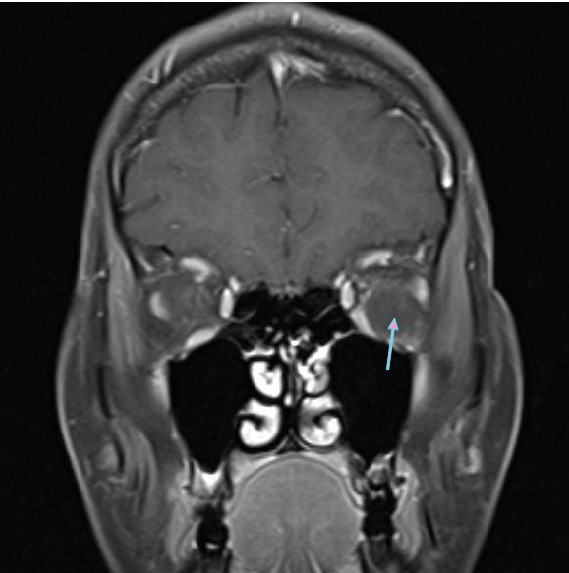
Coronal postcontrast T1 weighted MR image showing nonenhanced hypointense haematoma (arrow).
